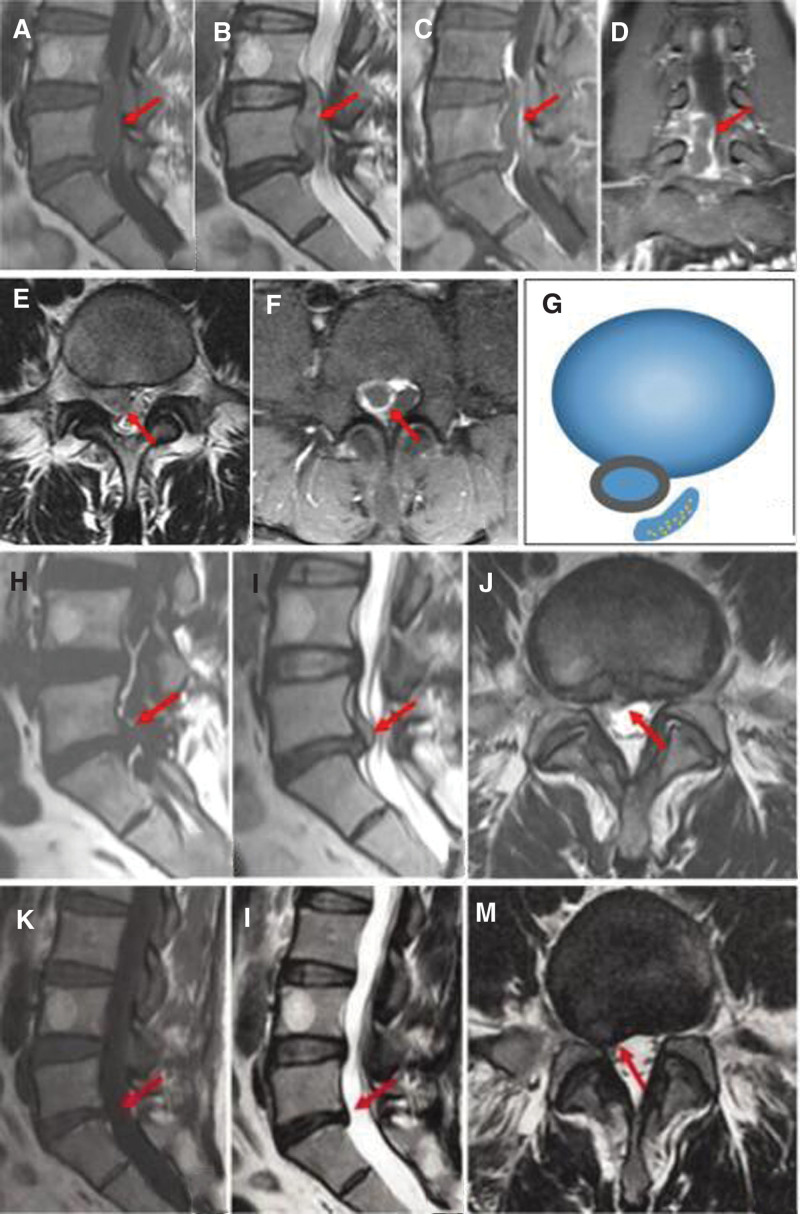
MRI images of a 43-years-old female with giant tumor-like LDH. (A, B, E) Plain MRI showing L_5_/S_1_ intervertebral disc sequestered protrusion with tumor-like changes. (C, D, F, G) On enhanced MRI, the sequestered nucleus pulposus can be seen with marginal annular enhancement and no enhancement centrally, suggesting a positive “bull’s eye” sign (Type A). (H, I, J) Plain MRI showing significant resorption of the protrusion. (K, L, M) Plain MRI showing complete resorption of the L_5_/S_1_ intervertebral disc protrusion, with a protrusion rate of 0% and a RR% of 100%. LDH = lumbar disc herniation, MRI = magnetic resonance imaging, RR% = resorption rate.
